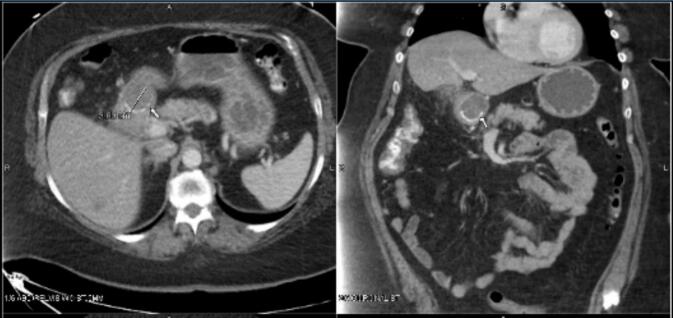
Patient 1's CT scan of the abdomen and pelvis showing large gallstone in the duodenum.
